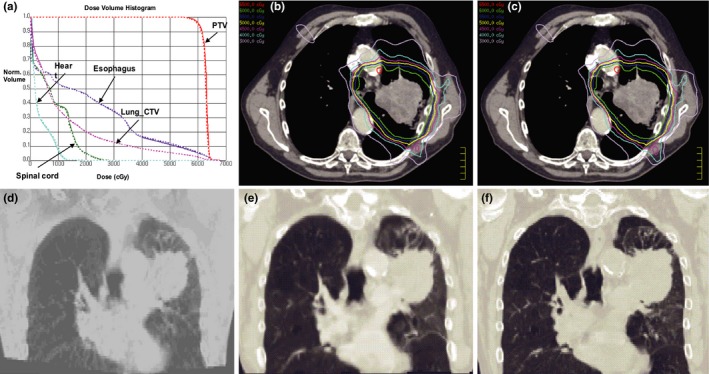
Another case exhibiting good agreement between vCT (dash line) and rCT (solid line) based accumulated doses (a), and isodose distribution comparison between vCT‐based accumulated dose (b) and rCT‐based accumulated dose (c), with their corresponding CBCT (d), vCT (e), and rCT (f). CBCT, cone beam CT.
